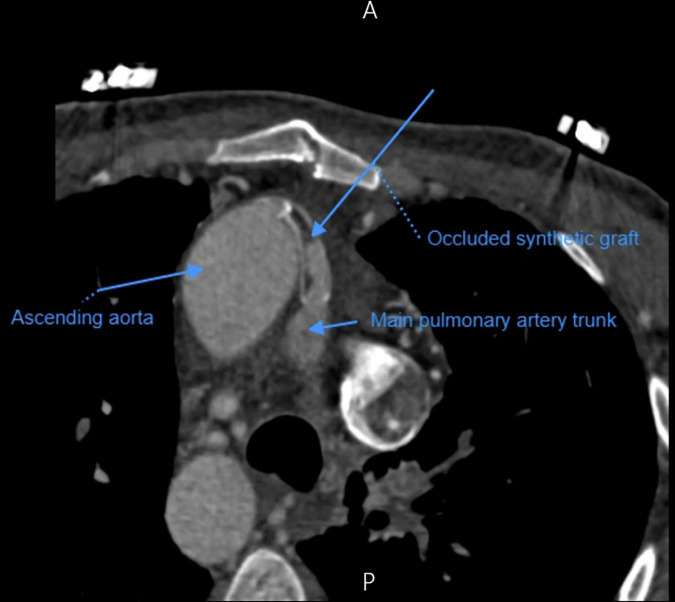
CT-scan showing occlusion of central aorto-pulmonary shunt.
